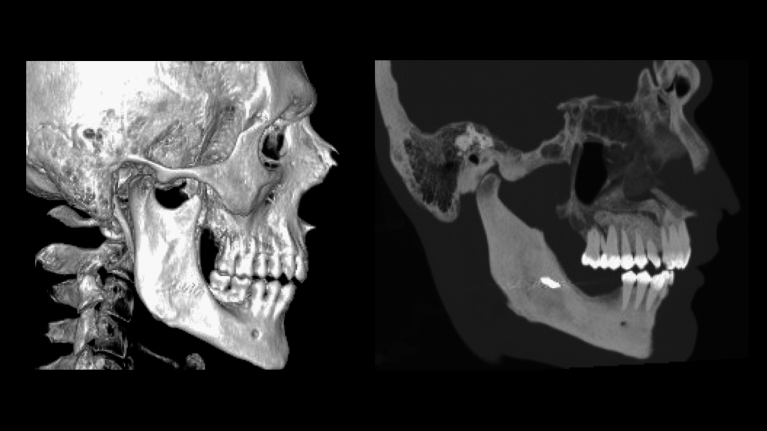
One year after the intervention, the CT showed complete resolution of the AVM and complete ossification of the right body of the mandible
